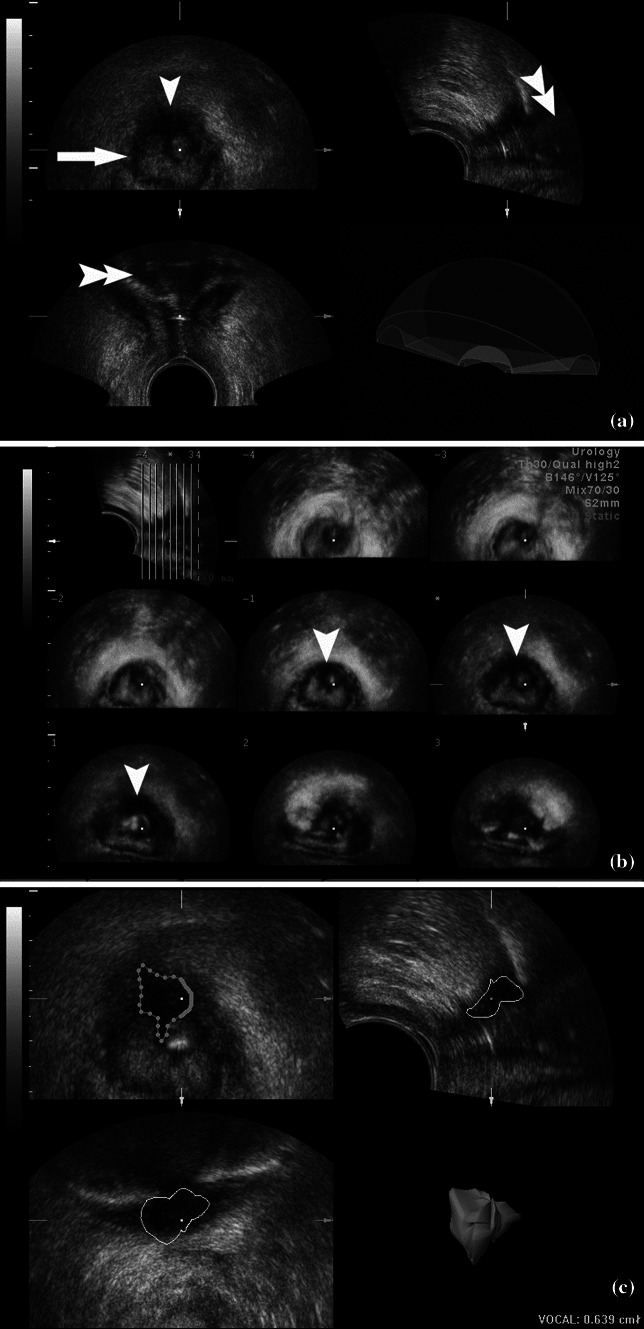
a Multiplanar reconstruction: on axial view (*upper left*), a thin circular (*arrow*) scar in the external anal sphincter and a bulky scar (*arrowhead*) that involves both sphincters and is adherent to the anoderm are seen. Short (1 cm long) obstruction with distended rectal ampulla (*double arrowheads*) is visible on sagittal (*upper right*) and coronal (*lower*) planes. b Tomographic ultrasound imaging with static volume contrast imaging. Bulky scar (*arrowheads*) on consecutive axial slices seen in the *upper* part of anal canal (see the *pilot sagittal image*—*upper left*). c Evaluation of volume of the bulky scar and its 3D presentation (*lower right*)
